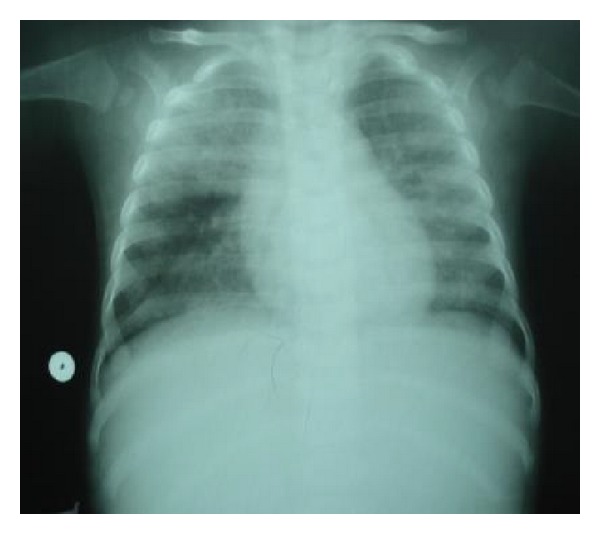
Initial chest X-ray: bilateral alveolointerstitial infiltrate.
